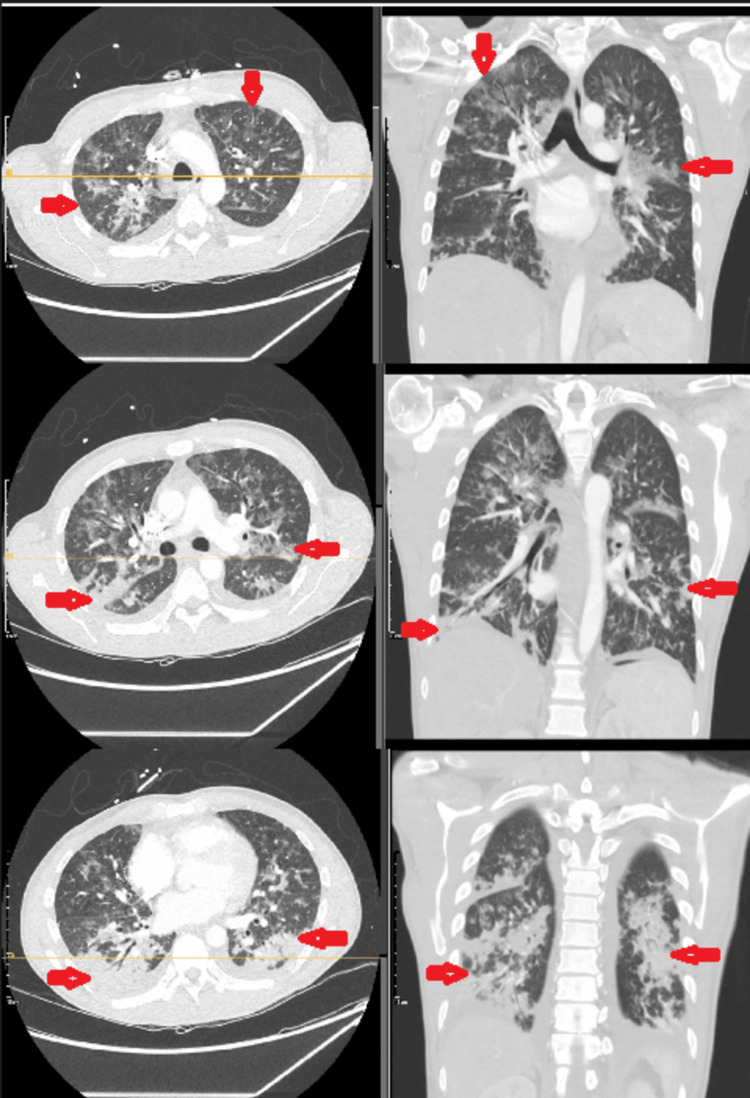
Axial and coronal views of chest CT angiography at three different levels, showing extensive bilateral consolidation, ground-glass, and nodular opacities (marked by red arrows)
